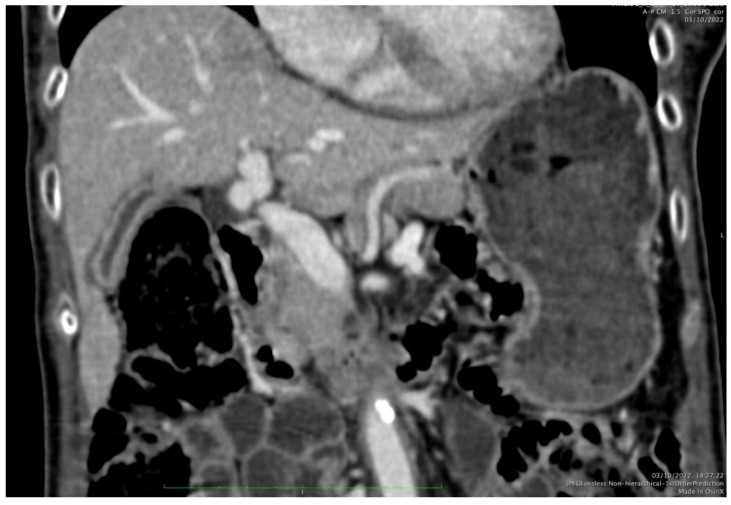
Abdominal CT with intravenous iodine contrast: peri-cystic fluid, inflammation, and wall thickening in a patient with abdominal pain while receiving Lenvatinib. Gallbladder stones were not identified.
